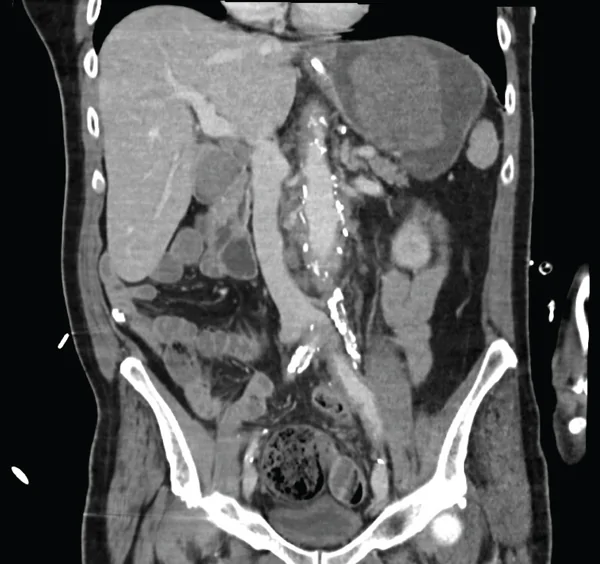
CTA of the abdomen and pelvis with large hyperdense intraluminal material in the stomach.
